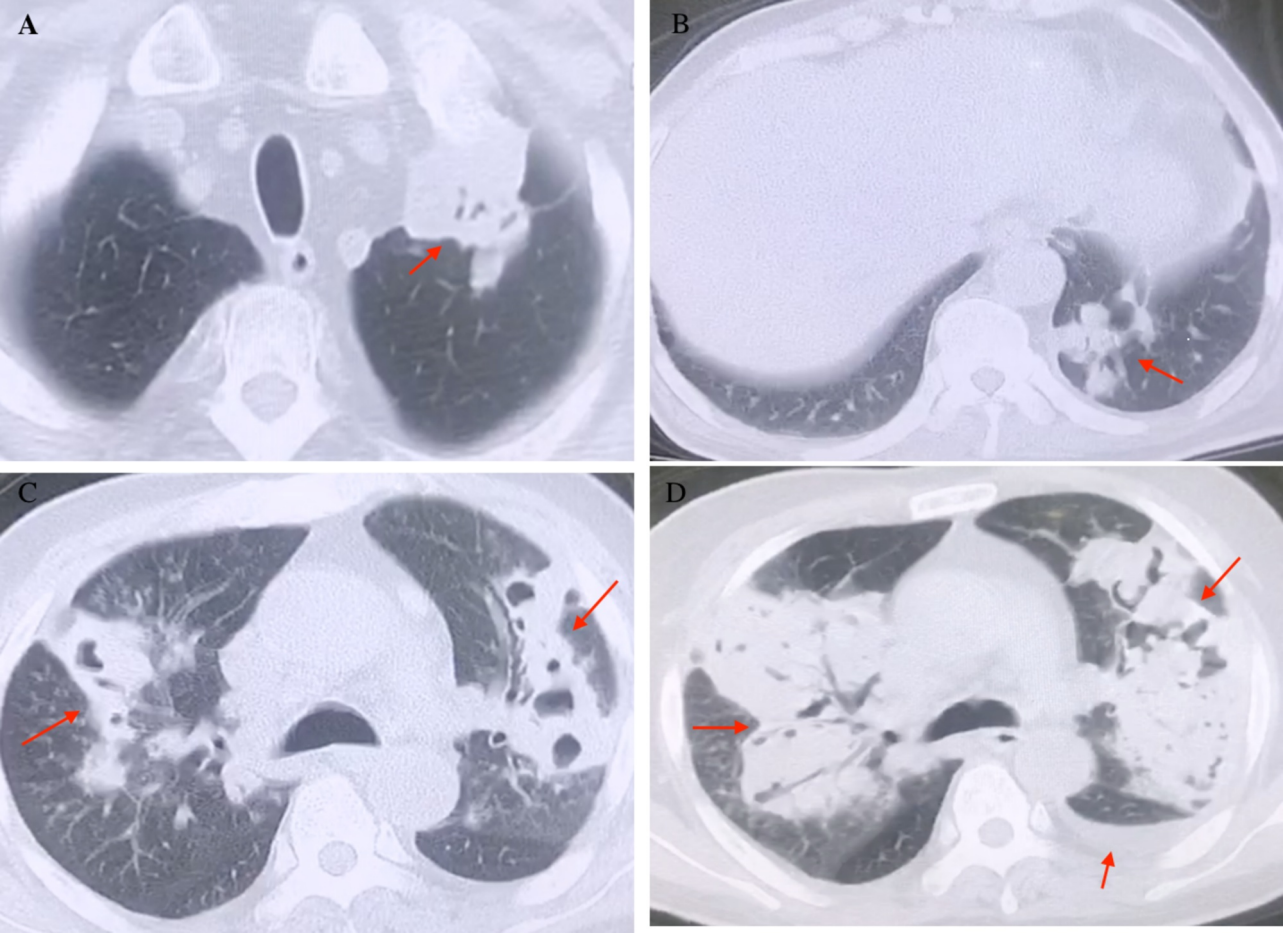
Chest CT of the patients. A: mass in the left upper lobe. B: multiple nodules in the left lower lobe. C: consolidation and multiple cavitations with air bronchogram in bilateral lobes. D: bilateral pulmonary diffuse lesions with pleural effusion
